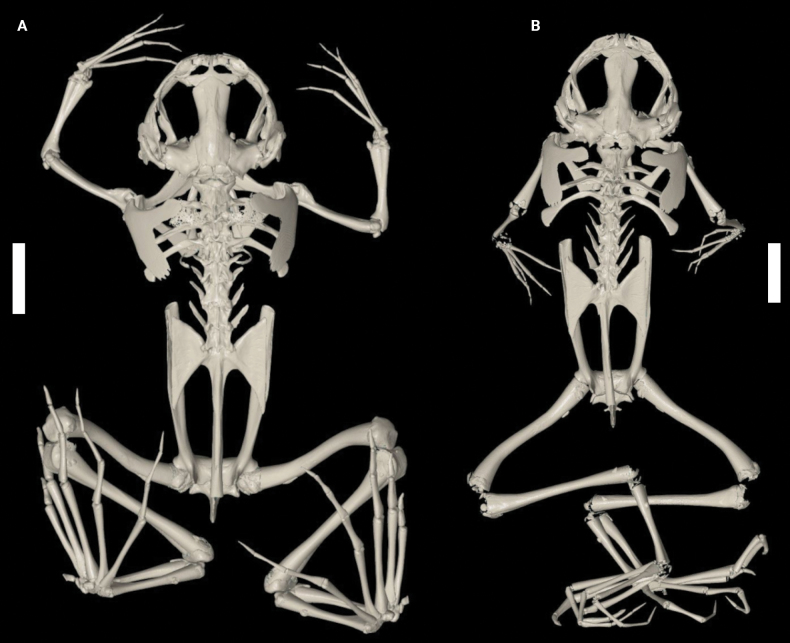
X-ray computed tomogram of the full skeleton of adult *Xenopuslaevis* (A male RBINS 18733 B female RBINS 18736) from Comines-Warneton, Belgium, in dorsal view. Scale bars: 1 cm.
